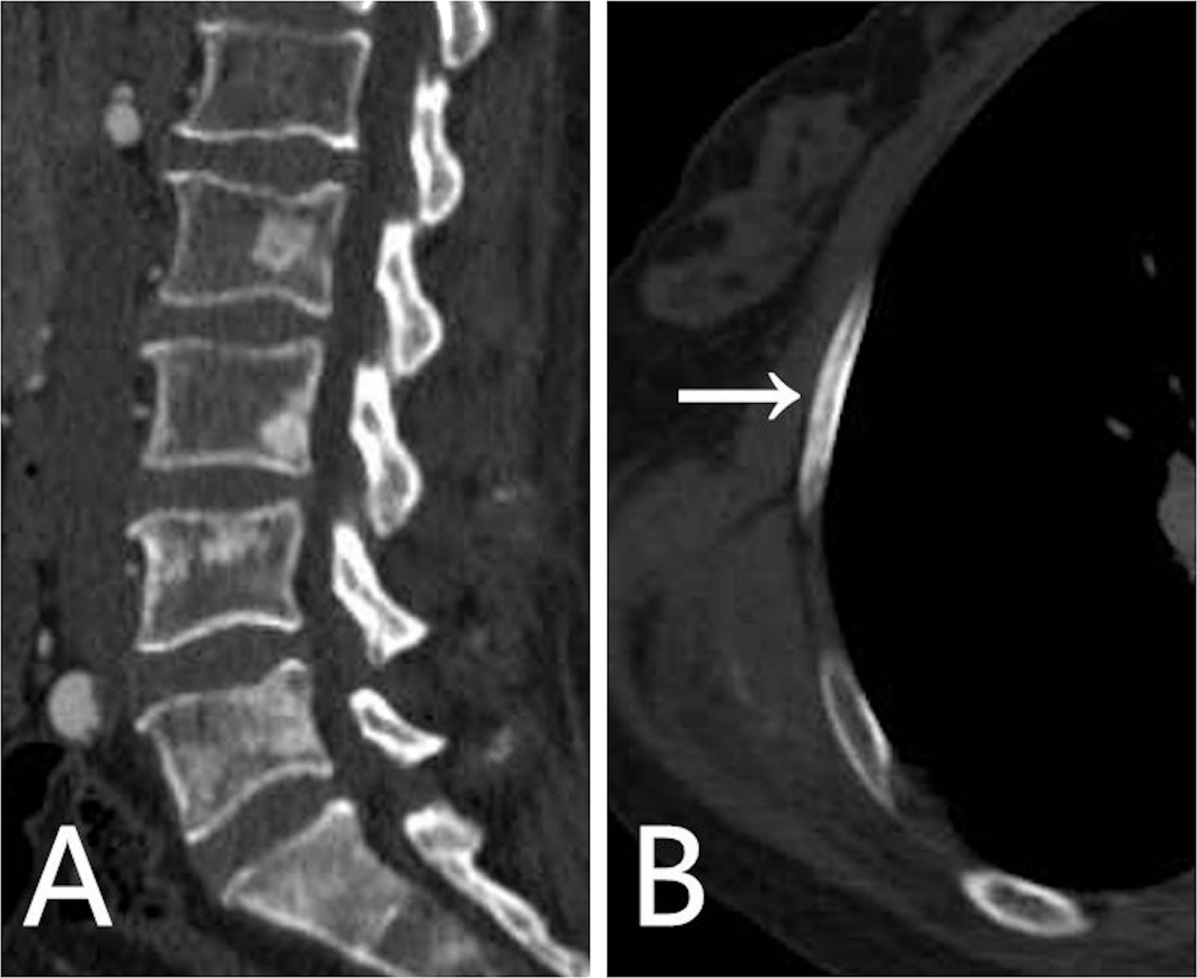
CT images in a 40-year-old woman with spine and ribs involved. A Sagittal reconstruction spine CT image shows heterogeneous diffuse osteosclerosis in the L-5 and S-1 vertebrae, and focal osteosclerosis in the L2-4 vertebrae. B Axial chest CT image shows patchy osteosclerotic lesion of the ninth right side rib without expansion (arrow)
